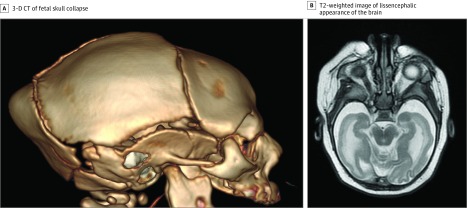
Neuroimaging Findings of an Infant With Severe Zika Virus A, Three-dimensional (3-D) computed tomography (CT) reconstruction demonstrates classic phenotypic pattern of fetal skull collapse with overlapping cranial sutures and prominent occipital protrusion. B, T2-weighted imaging demonstrates simplified gyral pattern with a lissencephalic appearance of the brain.
